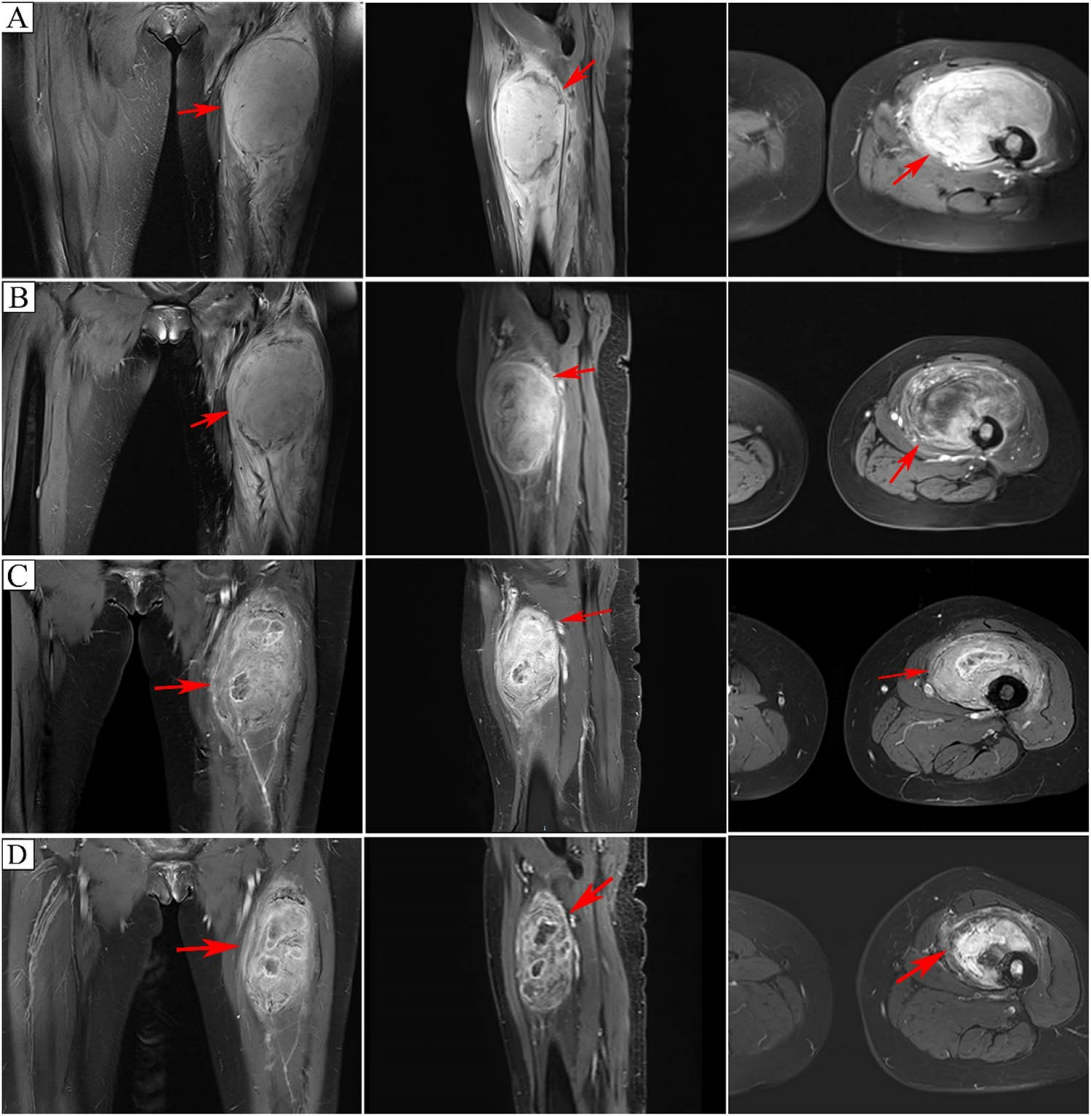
Lower limb MRI. A Pre-treatment(cadonilimab plus anlotinib), increased fat-suppressed signal in the upper left femur bone marrow, adjacent large mass (108 × 84 × 71 mm) with high signal intensity, distinct boundary, increased peripheral muscle signal, uneven enhancement, and anterior femoral cortex destruction. B Post-treatment, high signal mass in the upper thigh measured 100 × 85 × 61 mm. Anterior left thigh soft tissue mass decreased slightly; other results remained relatively unchanged. C After five treatment cycles, high signal mass in the upper thigh measured 92 × 76 × 53 mm. Compared to pre-treatment, the lesion diminished, with multiple central necrotic areas. D After seven treatment cycles, high signal mass in the upper thigh reduced to 78 × 66 × 45 mm
